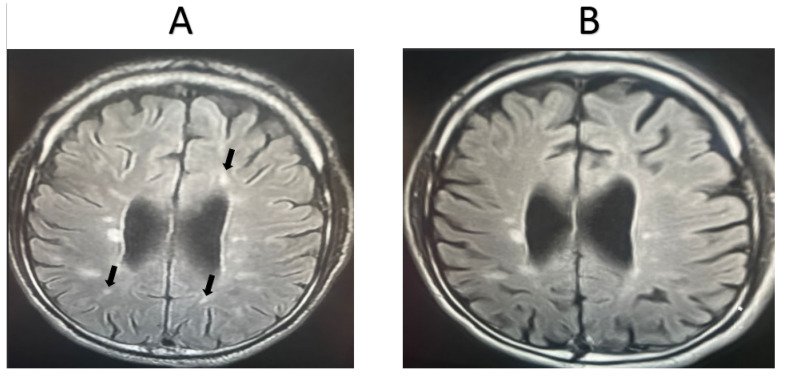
MRI examination of a second MSW patient taking fingolimod. (A). The number of lesions before treatment = 13, with an average lesion size (cm) of 0.48 ± 0.24, (B). The number of lesions after treatment = 10, with an average lesion size (cm) of 0.37 ± 0.20. The number and extent of lesions were similar after one year of fingolimod treatment (stable). Arrows refer to lesions.
